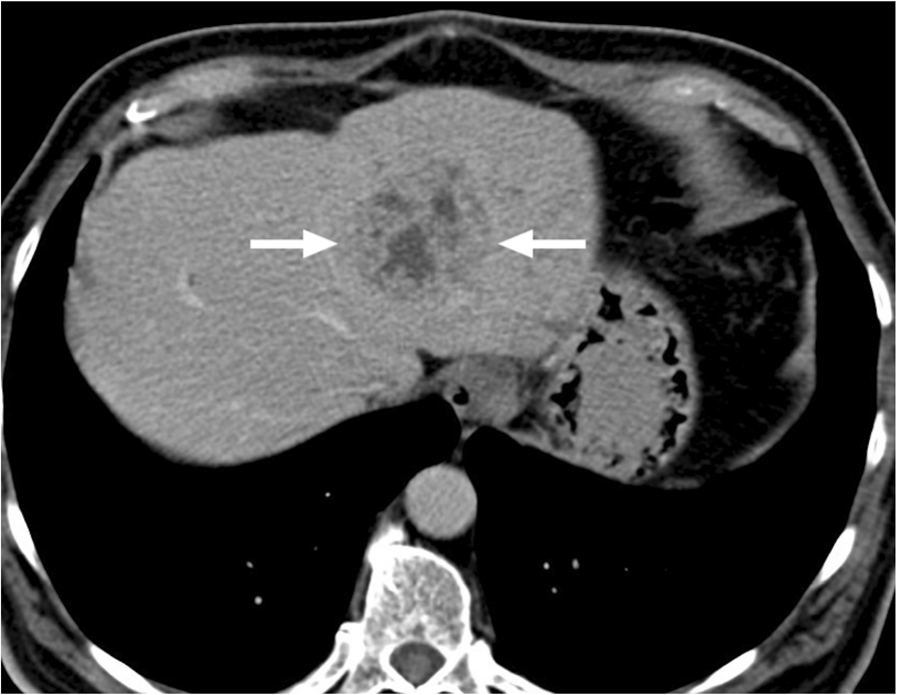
A 70-year-old male with known colon cancer under complete remission underwent a routine screening CT examination. Axial plane postcontrast CT image revealed 5 cm predominantly solid mass (arrows) within the left liver lobe. The patient was completely afebrile and free of any abdominal pain at the time of the CT scan. Percutaneous biopsy confirmed liver abscess with no evidence of neoplastic cells. Follow up CT scan (not shown) after IV antibiotics confirmed significant regression of the lesion
